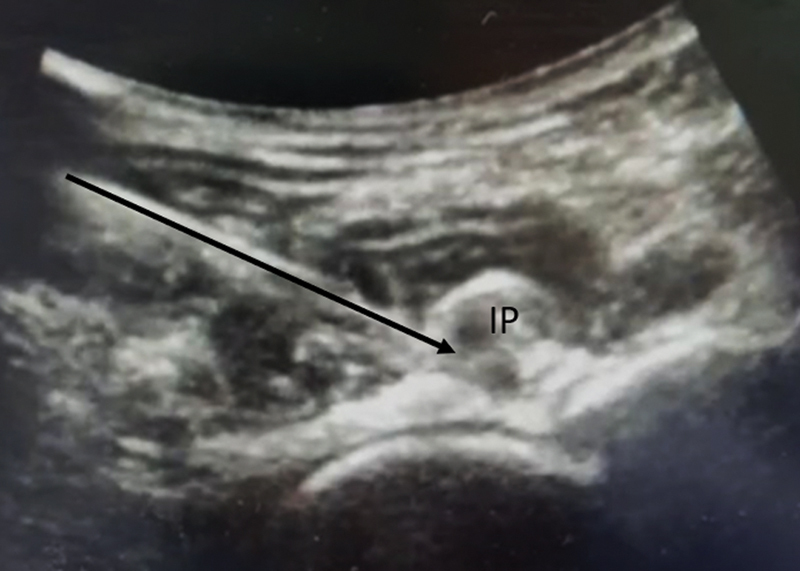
Ultrasound image of the blade path during guided iliopsoas tenotomy.
